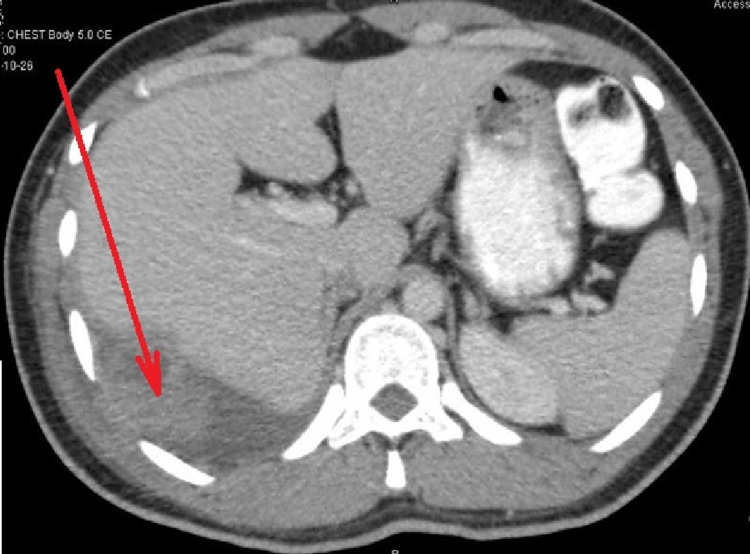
CT scan of the chest, abdomen, and pelvis. It shows that the lesion is seemingly invading the lower posterolateral pleura (red arrow).
